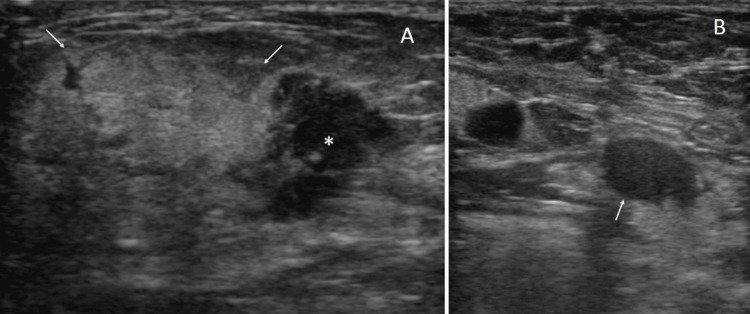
Ultrasound findings Ultrasound showed widespread, thick hyperechoic areas with indistinct margins (arrows), hypoechoic areas (asterisk) (A), and a lymph node with a diffuse punctate hyperechoic pattern (arrow) in the axilla (B).
